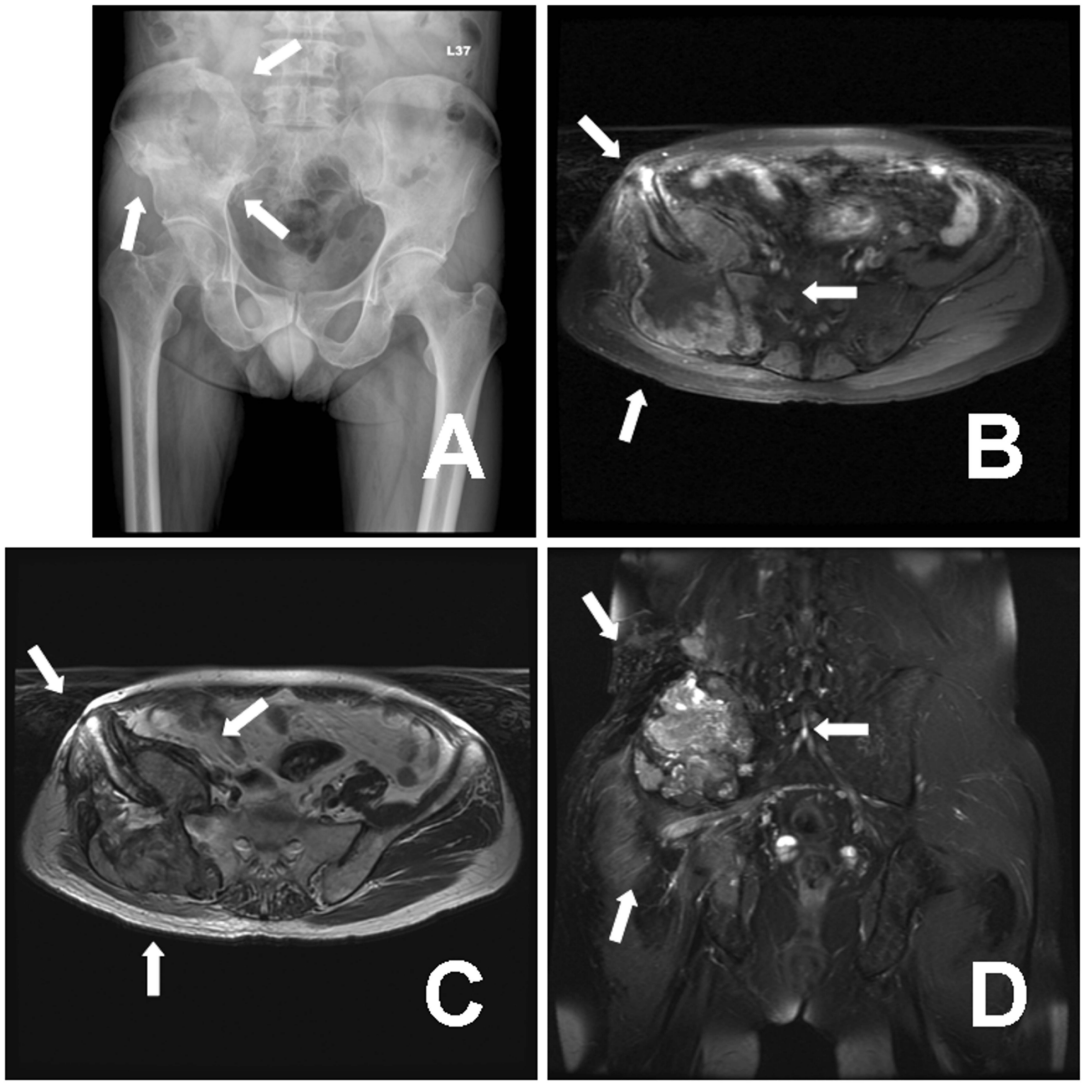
Pelvis radiograph showed a large ill-defined osteolytic lesion at right iliac bone accompanied with iliac wing fracture (white arrows) (A). Repeated MRI revealed a 9.4 × 9.2 × 9.0 cm bipeduncular lesion arising from previous operation site, accompanied with iliac wing fracture (white arrows) (B to D). B: axial fast spin echo (FSE) fat-suppressed T1-weighted image with contrast enhancement. C: axial fast recover fast spin echo (frFSE) T2-weighted image. D: coronal fat-suppressed (FS) FSE T2-weighted image with contrast enhancement.
